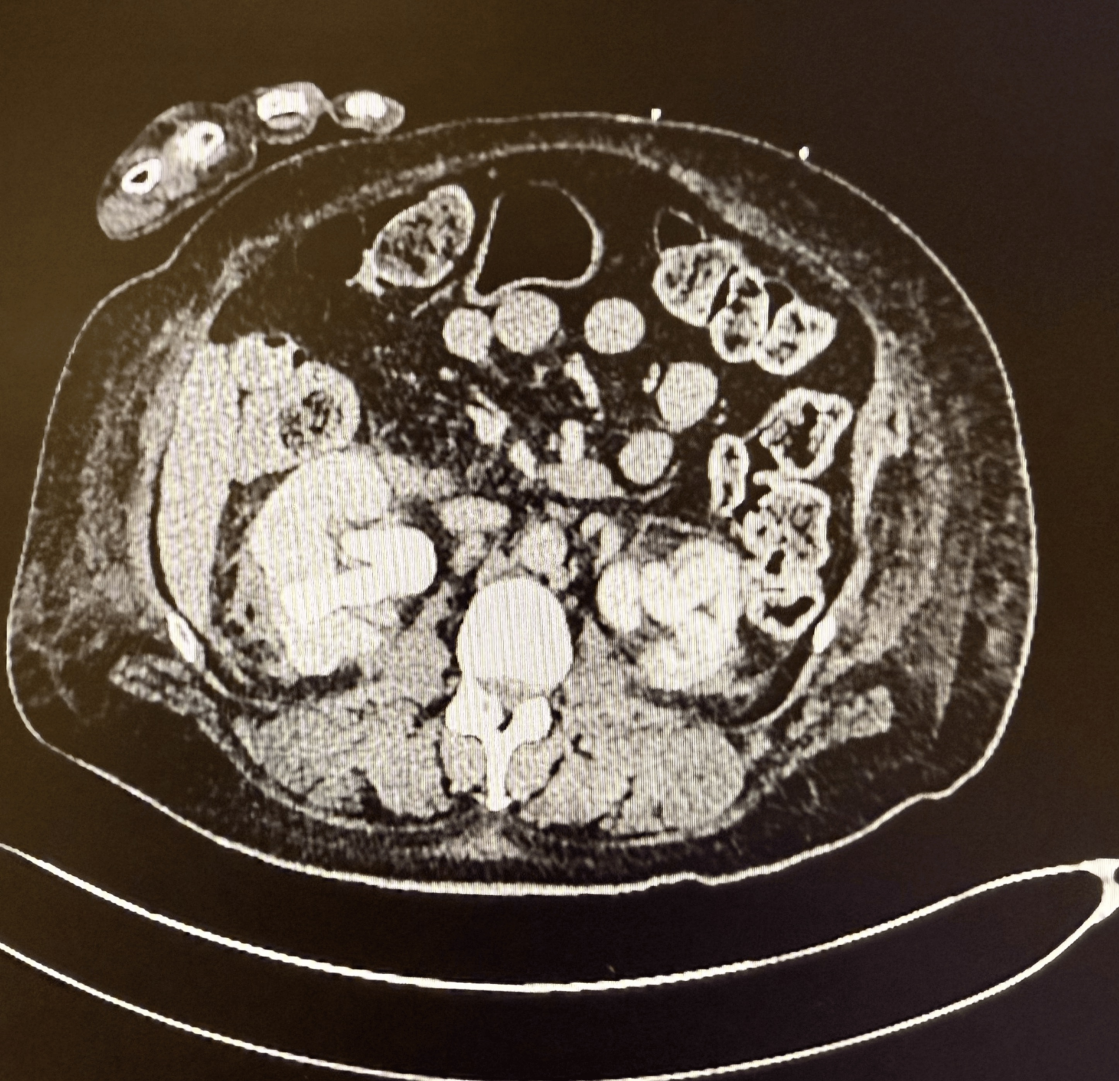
The CT urogram showed right perinephric stranding; no bleeding source was noted.
